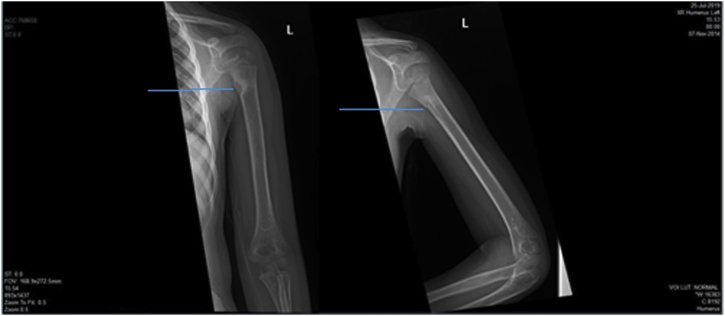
Case 2, plain x-ray left upper limb of the older girl revealed green-stick fracture of medial cortex left humerus surgical neck (blue lines).
